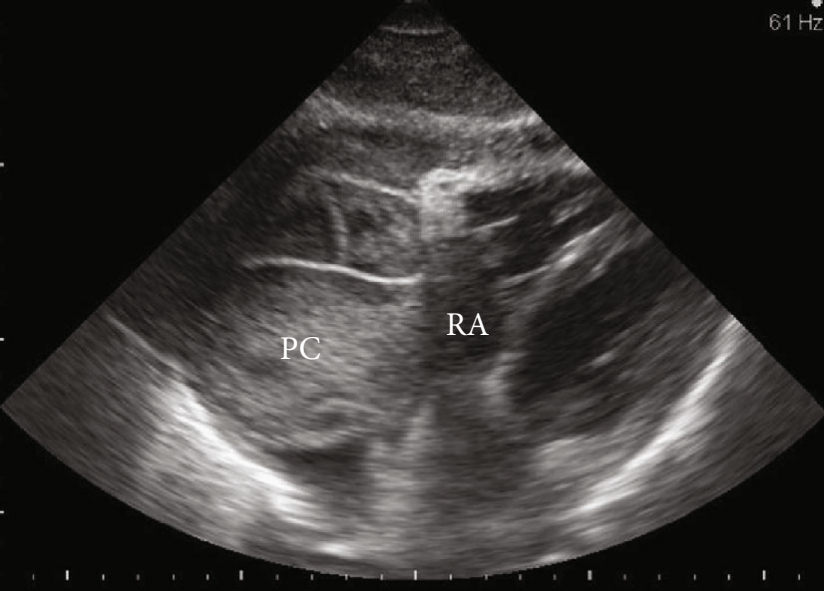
Echocardiography—subcostal four-chamber view. PC—encapsulated pericardial effusion with fibrinous septa compressing the right atrium (RA).
